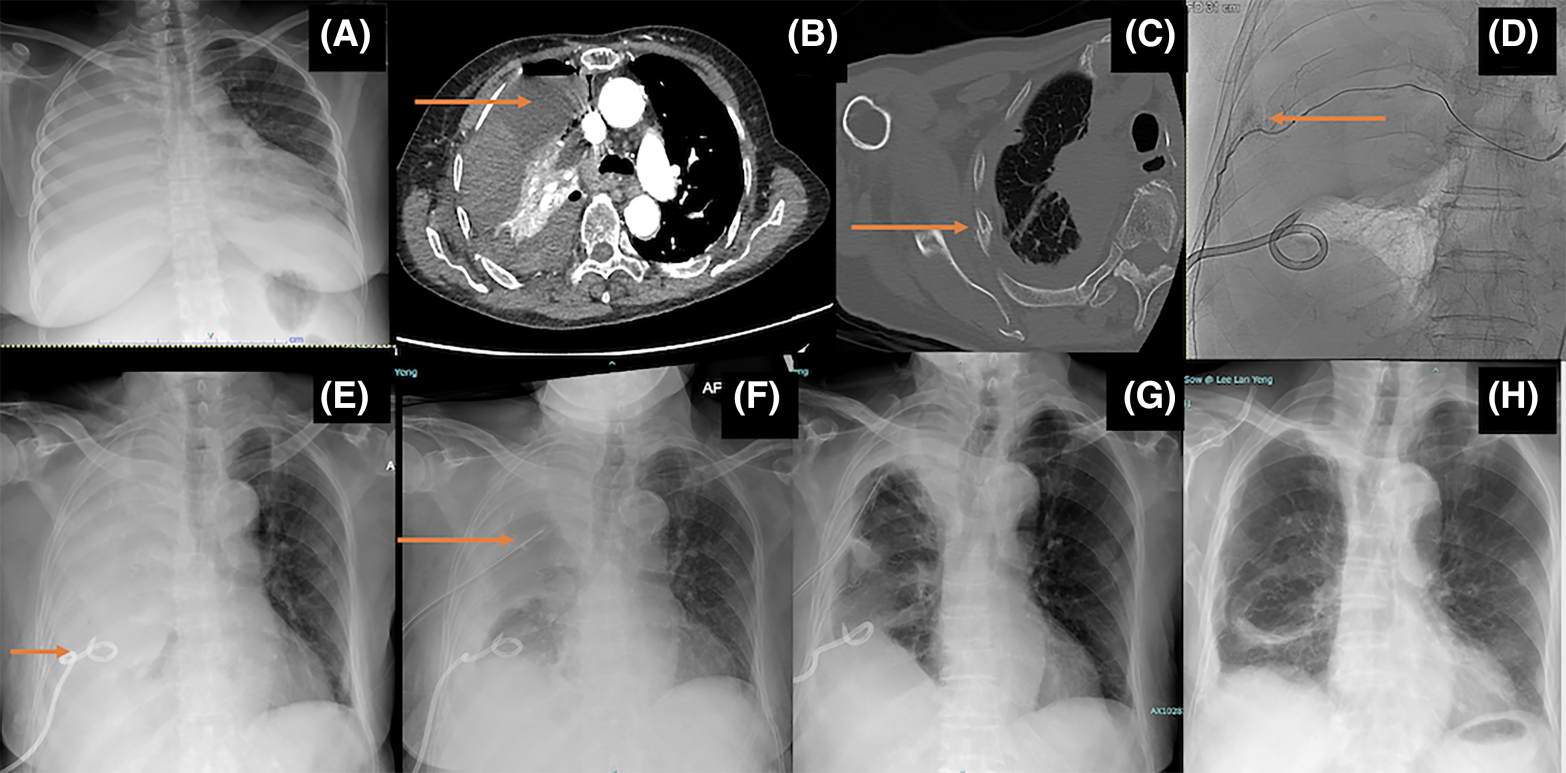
Chest radiograph (A) on arrival revealed massive right pleural effusion. Four contrast enhanced computed tomography (CECT) Thorax (B) revealed heterogenous hyperdensity of pleural fluid (red arrow) consistent with hemothorax. CECT Thorax (C) revealed right 7th rib fracture. CT Thoracic angiogram (D) revealed bleeding right 7th intercostal artery. Chest radiograph (E) revealed ICC in‐situ (red arrow) with persistent hemothorax. Chest radiograph (F) post large bore chest tube (red arrow) showed slight reduction of right hemithorax opacity. Chest radiograph (G) post 4 cycles of intrapleural alteplase showed significant radiological improvement. Chest radiograph (H) one month post discharge revealed resolution of hemothorax.
